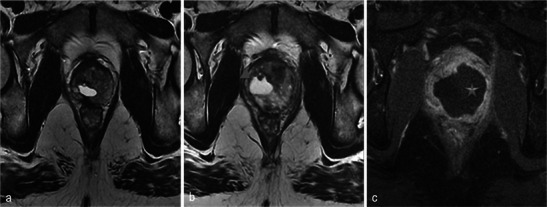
Contralateral treatment. A 56-year-old patient, with initial right photodynamic therapy, then left therapy in a second intervention. Pre-left therapy (a) and day-7 post-left therapy (b) axial T2-weighted images; day-7 post-left therapy (c) axial T1 FS contrast-enhanced MR image. On the left lobe, a heterogeneous T2 area with low signal in T1-weighted image, unenhanced, corresponding to the recent tissue necrosis (*star*). In the right lobe the T2 hyperintense old cystic cavity increased in size (*arrowhead*)
